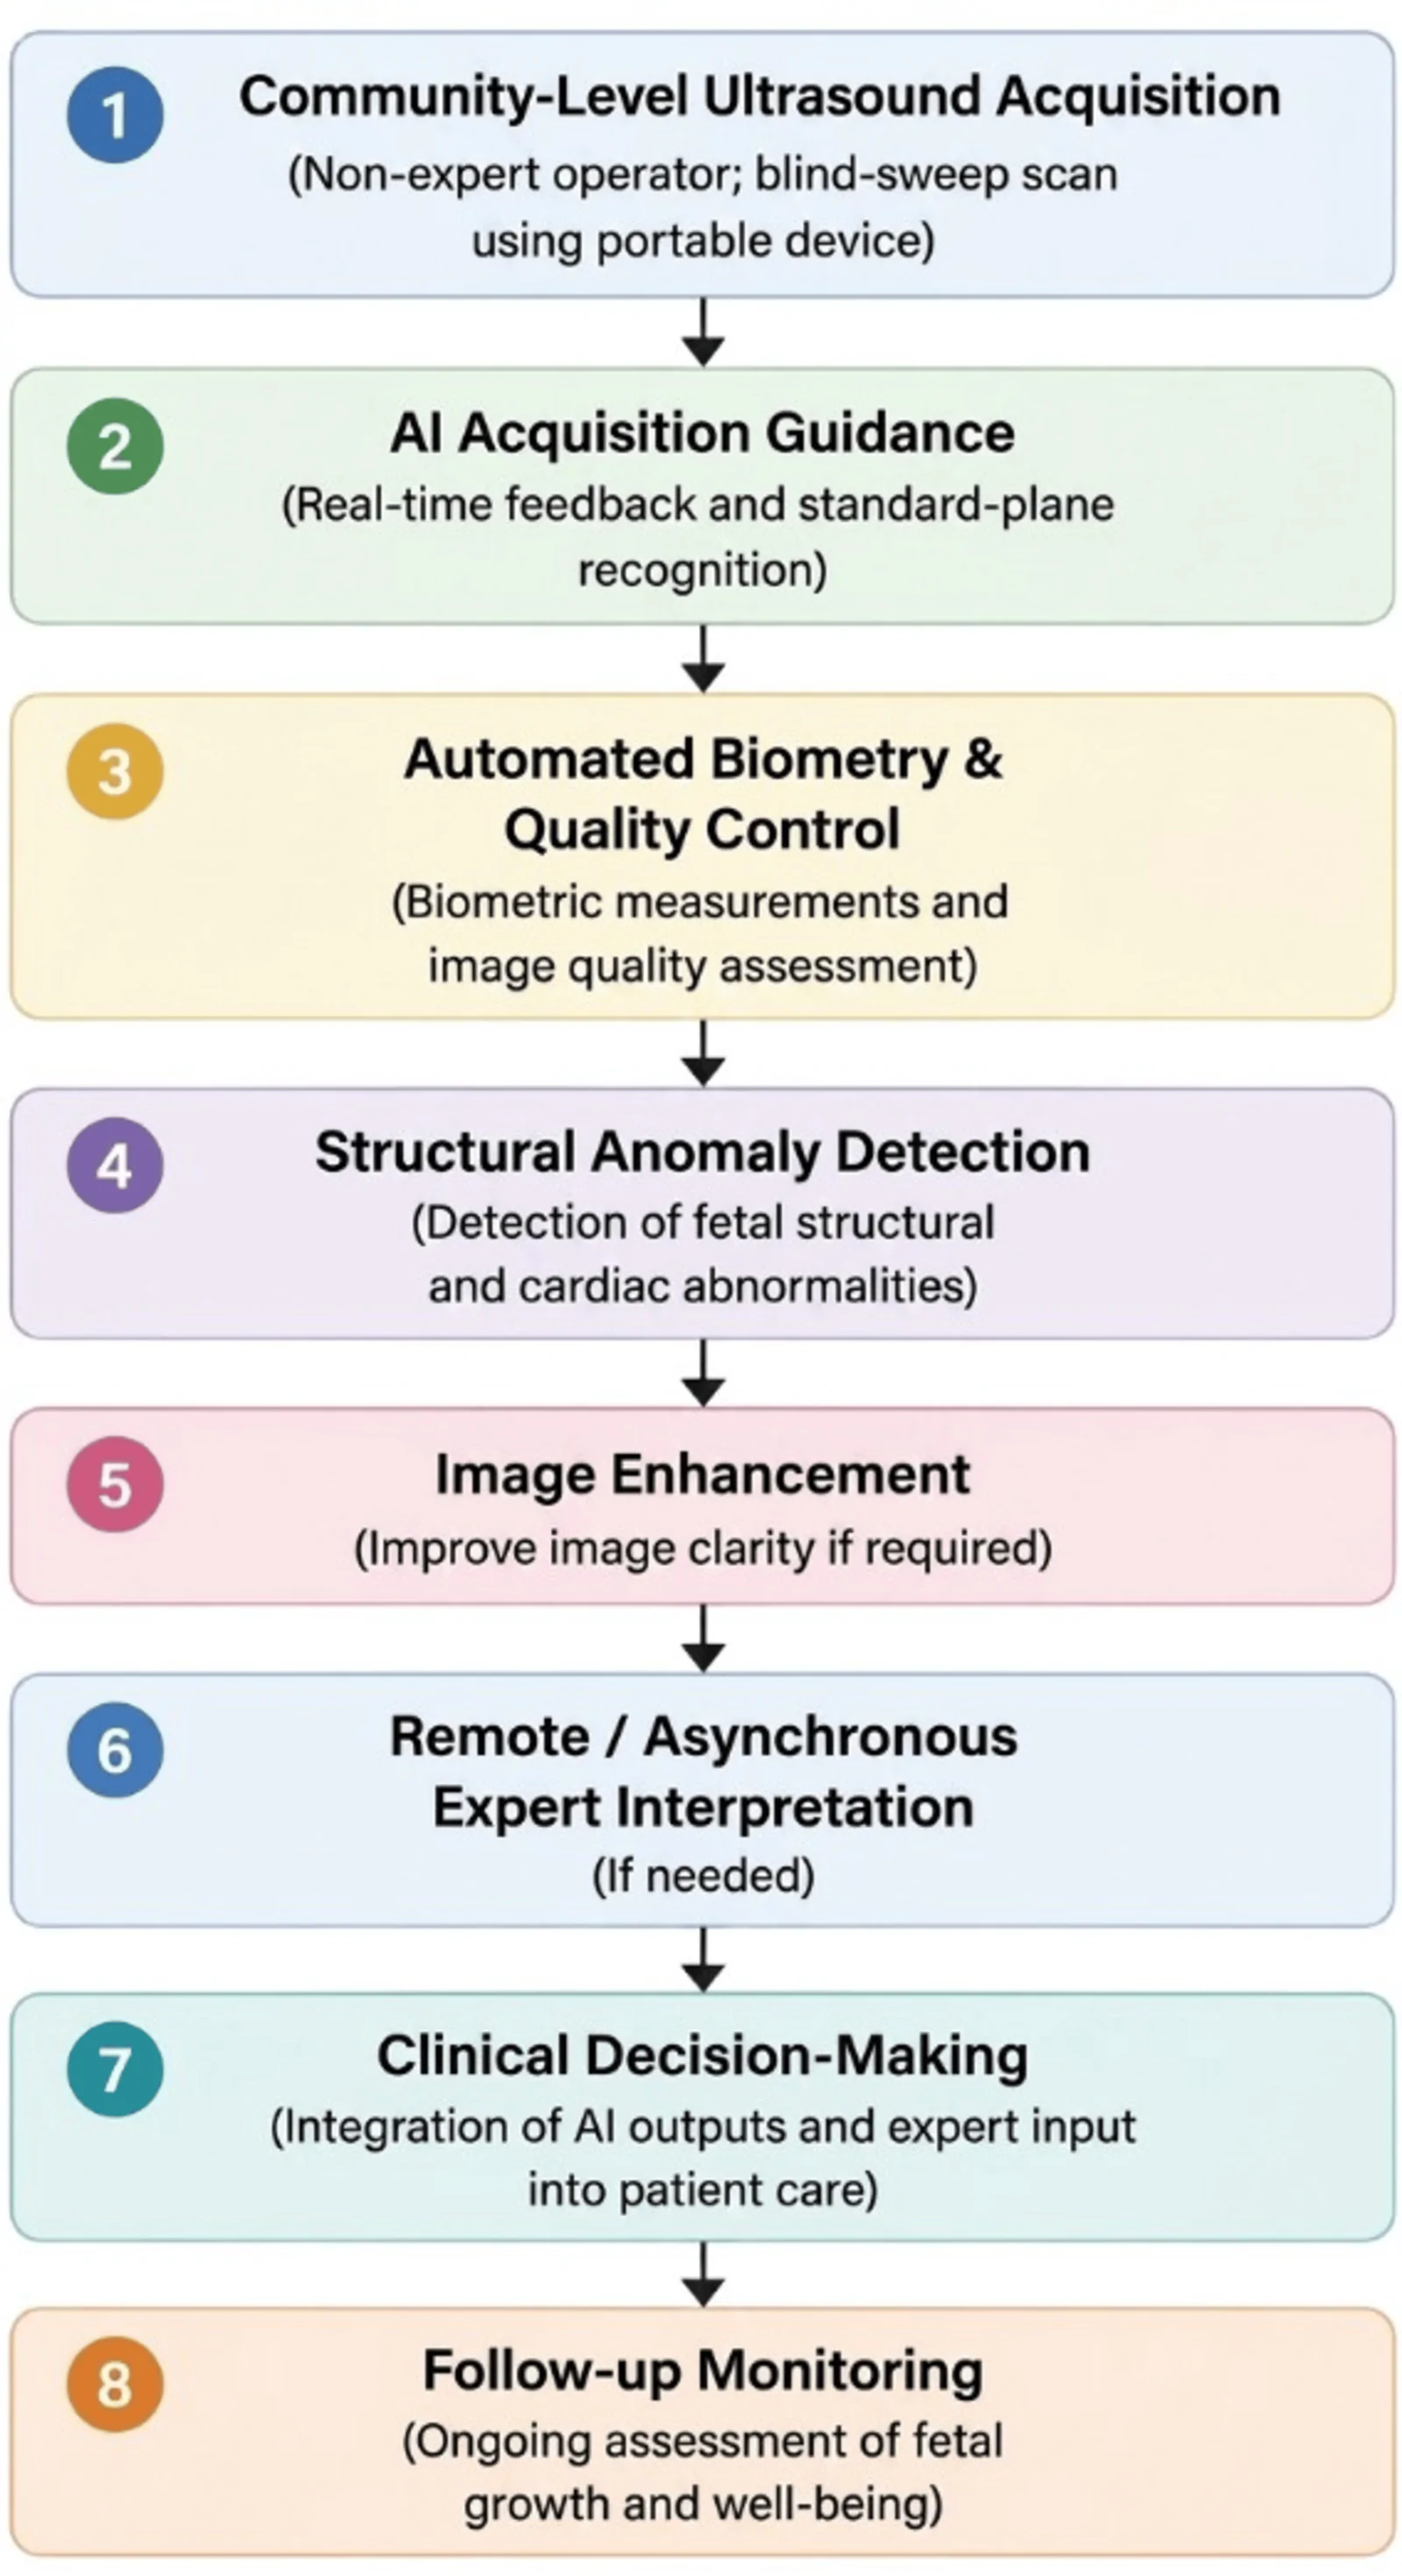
AI-assisted fetal ultrasound care pathway in low-resource settings Original figure created by the authors using https://whimsical.com/ (Whimsical, Denver, Colorado, US)
